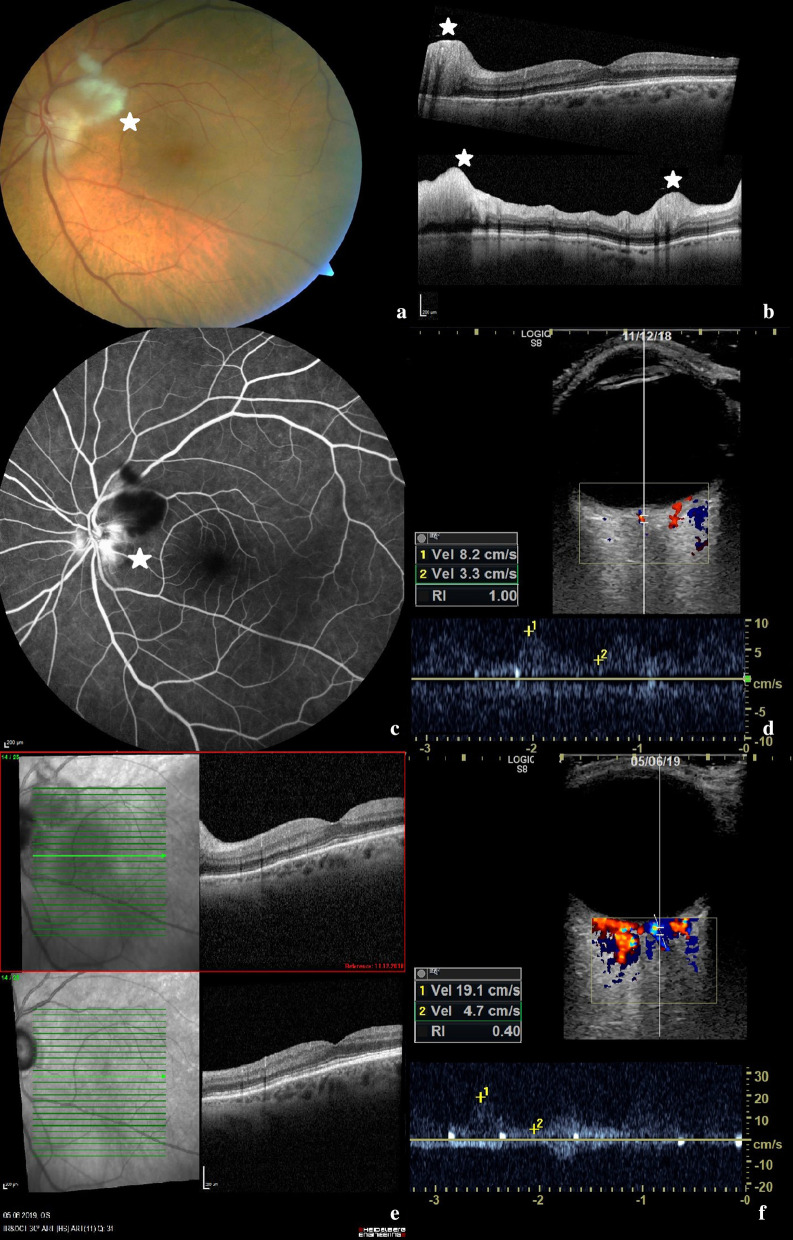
Ophthalmologic imaging and transocular ultrasound of the left eye. a Colour fundus retinal photography of the left eye displaying an optic disc edema with adjacent cotton wool spot (*) along the upper temporal vascular arch. b Macula-optical coherence tomography of the left eye with a normal macula and segmental disc edema. Retinal nerve fibre layer thickness was increased in the superior, temporal and inferior segments (*). c Fluorescein angiography of the left eye with distinct hyperfluorescence of the papilla, i.e. a “hot disc”. d Transocular ultrasound including measurement of flow velocity of the central retinal artery of the left eye on admission. e Macula-optical coherence tomography on admission (top) compared to 6-month follow-up (bottom). f Transocular ultrasound including measurement of flow velocity of the central retinal artery of the left eye in 6-month follow-up
